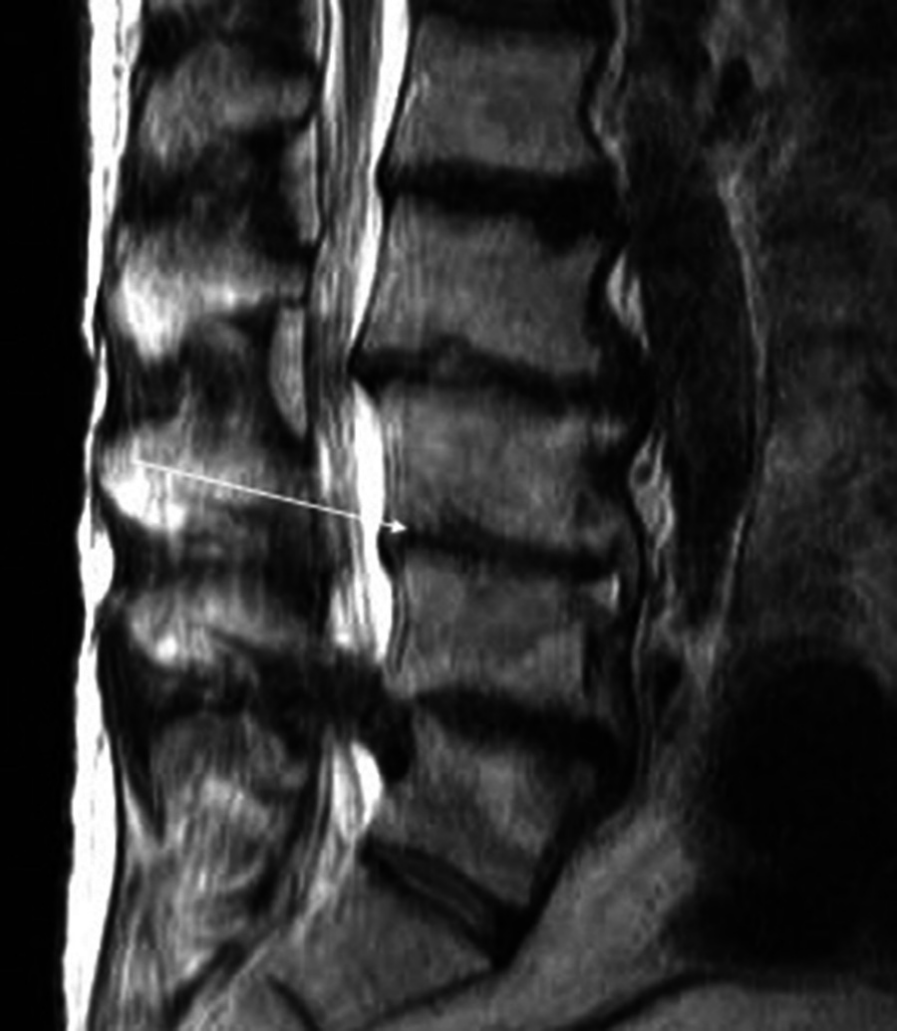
MRI without contrast. This image was taken nearly a year after he was discharged from the hospital. The anterior edema in the endplate is no longer present on the T2 MRI scan. The patient is asymptomatic with no signs of infection.
